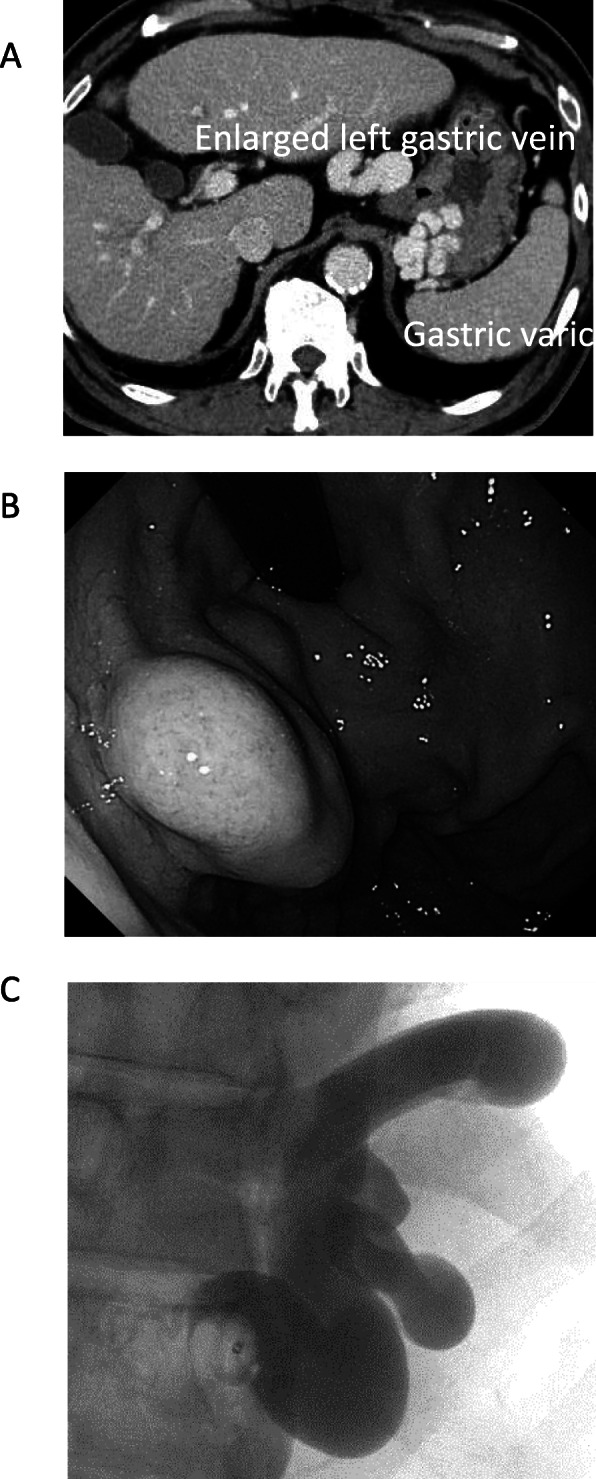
Imaging of gastric varices. a Gastric varices were detected by CT as an enlarged gastrorenal shunt from the left renal vein to the left gastric vein. b Gastric varices developed into enlarged nodules shown by upper esophagogastroduodenoscopy. CT, computed tomography
